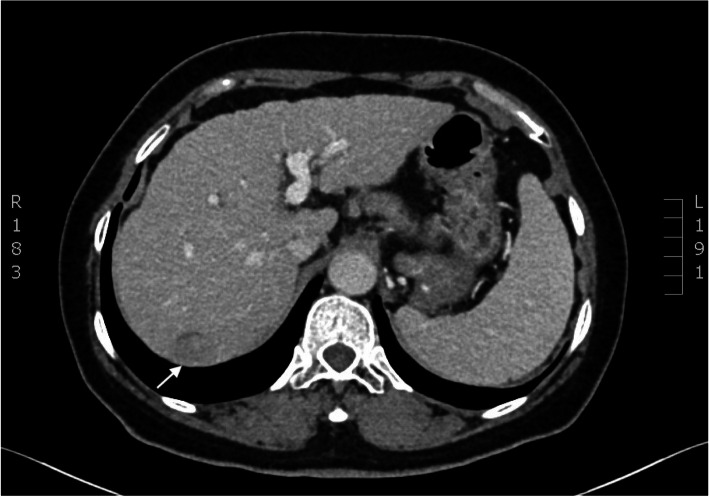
Enhanced CT showed a mass in segment VII of the liver with marked enhancement in the arterial phase and attenuation in the portal and delayed phases, presenting as a “fast in and fast out” pattern
